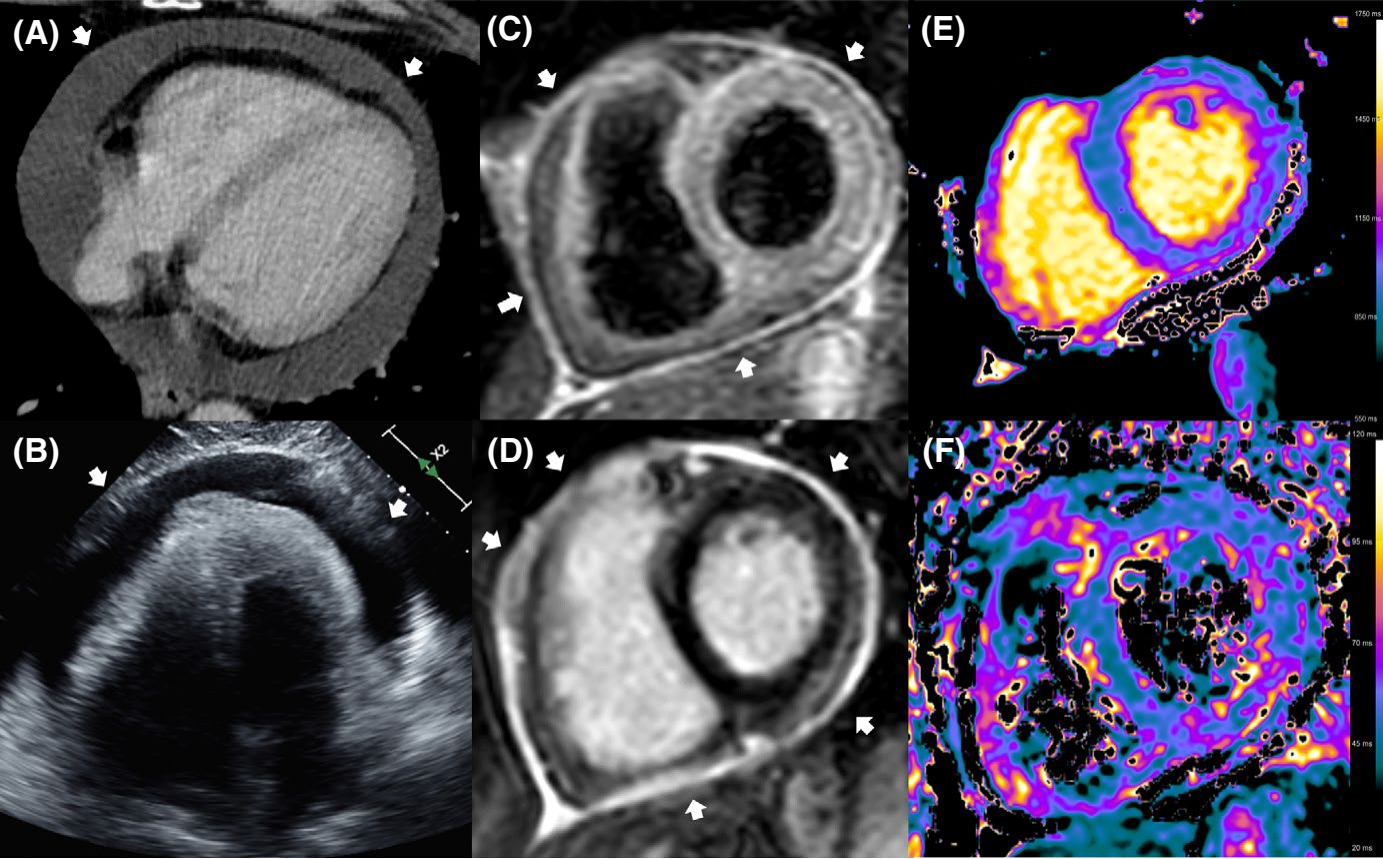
Panel A: Horizontal long‐axis view on an axial slice from cardiac computed tomography with a circular pericardial effusion (arrow). Panel B: Apical four‐chamber view from transthoracic echocardiography with huge circular pericardial effusion (arrow). The end‐diastolic diameter in front of the right ventricle is 28 mm. Panel C: Short‐axis black‐blood T2‐weighted view from cardiac magnetic resonance imaging (MRI) with pronounced pericardial edema (arrow). Panel D: Short‐axis T1‐weighted delayed contrast view from cardiac MRI presents a circular pericardial enhancement (arrow) without any myocardial texture changes (star). Panel E: Representative image of short‐axis native T1 mapping (identifying myocardial fibrosis) from cardiac MRI with normal myocardial texture. Global T1 relation time of 930 ms (center specific cut‐off value for acute myocarditis: >1000 ms). Color scale bar on the right‐hand side. Panel F: Representative image of short‐axis native T2 mapping (identifying myocardial inflammation) from cardiac MRI with normal myocardial texture. Global T2 relaxation time of 54 ms (center specific cut‐off value for acute myocarditis: 55.9 ms). Color scale bar on the right‐hand side
